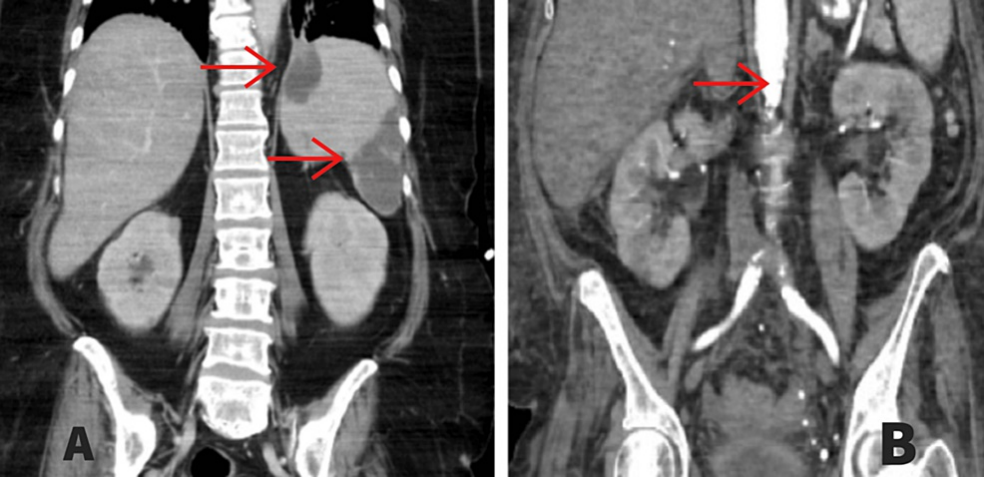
Computed tomography of the abdomen and pelvis. A: Septic emboli of the spleen. B: Septic emboli of the left kidney.
